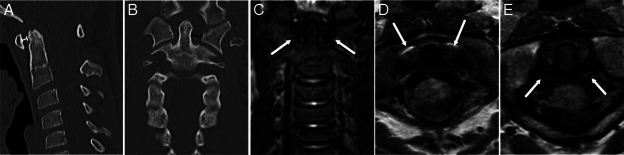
A 10-year-old male fell 2 m head first and reported severe neck pain. The atlantodental interval was reported as slightly widened (3.2 mm, capped white line), and the left-sided odontoid-lateral mass interval was also noted as widened (5.5 mm, capped black line). However, on magnetic resonance imaging (MRI), no edema is seen around the odontoid [image (C), white arrows] and the alar ligaments [image (D), white arrows] and the transverse ligament [image (E), white arrows] are intact. (A) Noncontrast computed tomography (CT), sagittal plane, (B) Noncontrast CT, coronal plane, (c) MRI, short tau inversion recovery, coronal plane, (D) MRI, proton density, axial plane, and (E) MRI, proton density, axial plane.
